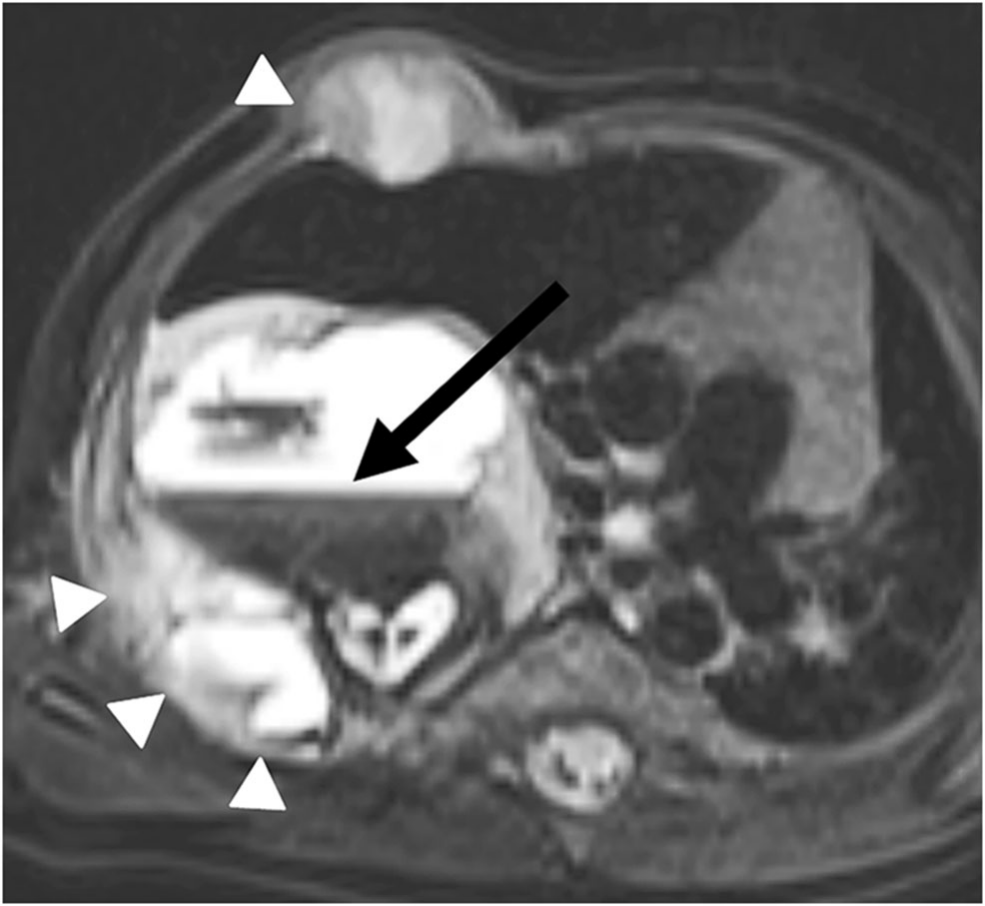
Multifocal mesenchymal hamartoma on lung MR in a 1-year-old boy. Axial fat-suppressed T2-weighted-turbo spin-echo image (MR parameters in Table 2) shows a multifocal mesenchymal hamartoma of the right thoracic wall with a large intrapulmonary portion in a newborn. The tumor originates primarily from the ribs (*arrowheads*), but manifests intrapulmonally. Blood-liquid levels (*arrow*) are a typical sign of associated aneurysmal bone cysts
